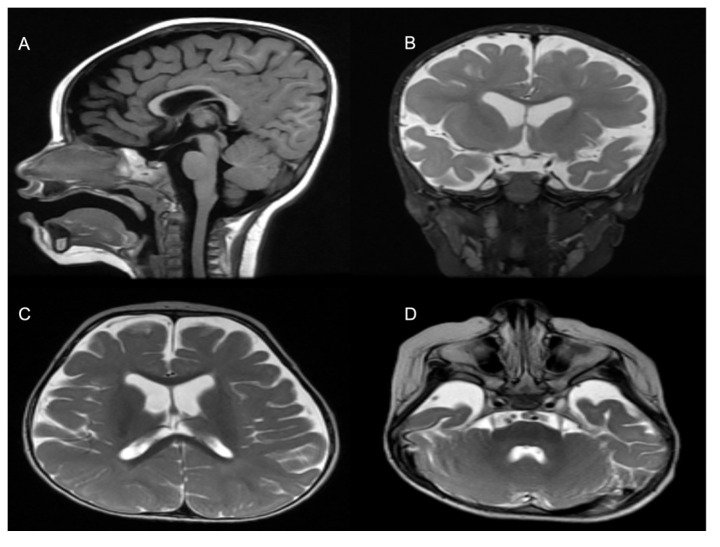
MRI Findings. (A) Mild thinning of the corpus callosum. Selected coronal T2 (B) and axial T2-weighted images (C,D) show volume loss of the cerebral hemispheres with resultant mild expansion of the lateral ventricles and CSF spaces.
